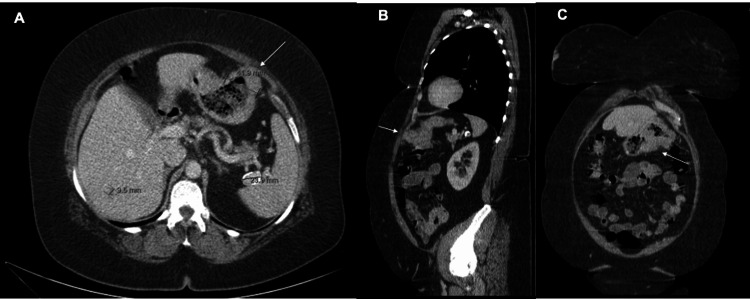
Contrast-enhanced abdominal CT scan showing the gastric lesion. (A) Axial view revealing an exophytic gastric mass measuring approximately 41.9 mm originating from the greater curvature, with irregular borders and surrounding fat stranding (white arrow). A concurrent 9.5 mm hepatic lesion is also identified, consistent with a hemangioma. (B) Sagittal reconstruction demonstrating the mass projecting anteriorly from the gastric wall. (C) Coronal view confirming the extraluminal growth pattern and spatial relationship with adjacent structures (white arrow).
